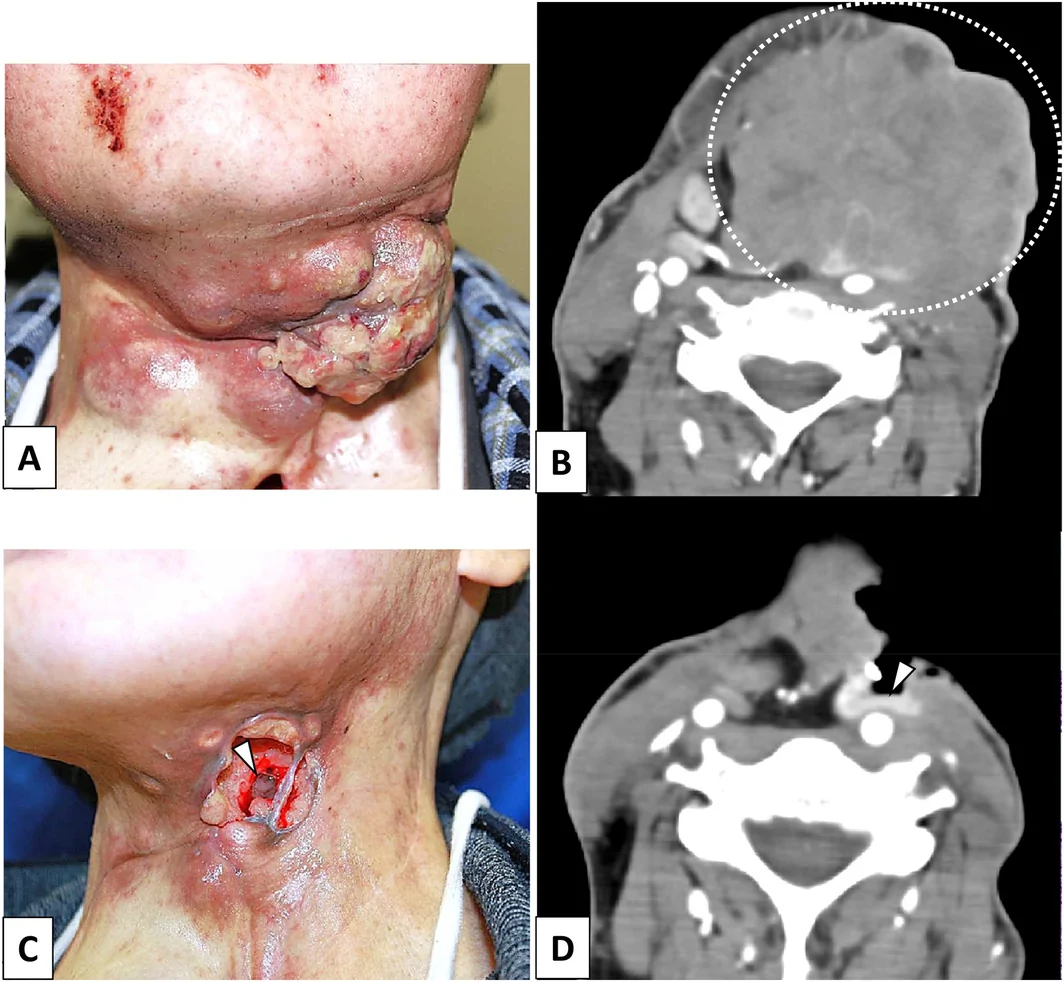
Therapeutic response to alternating VDC/IE chemotherapy. Before initiation of VDC/IE therapy. (A) Macroscopic appearance showing extensive cutaneous invasion and rapid tumor growth. (B) Contrast‐enhanced CT demonstrating a massive tumor infiltrating reconstructed tissues and lateral cervical structures (white dotted circle). After completion of two cycles of VDC/IE therapy: (C) Macroscopic view showing marked tumor shrinkage with formation of a large pharyngocutaneous fistula (white arrow). (D) Contrast‐enhanced CT confirming substantial tumor regression and the presence of the pharyngocutaneous fistula. The white arrow indicates the enhancing posterior wall of the remaining free jejunal graft. CT, computed tomography; IE, ifosfamide and etoposide; VDC, vincristine, doxorubicin, and cyclophosphamide.
